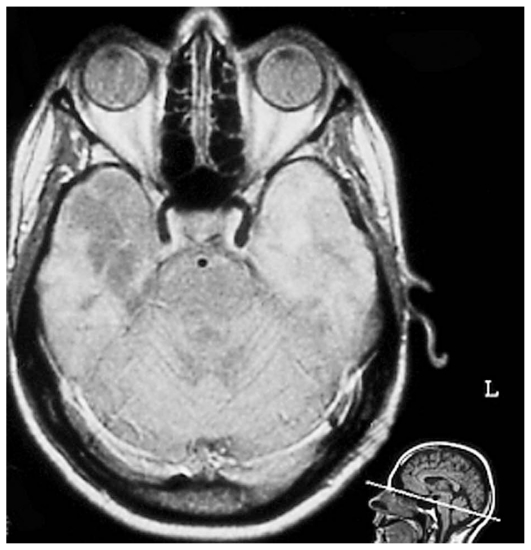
Herpes encephalitis, MRI section shows bilateral hyperdense lesions in the temporal and parietal lobes.
